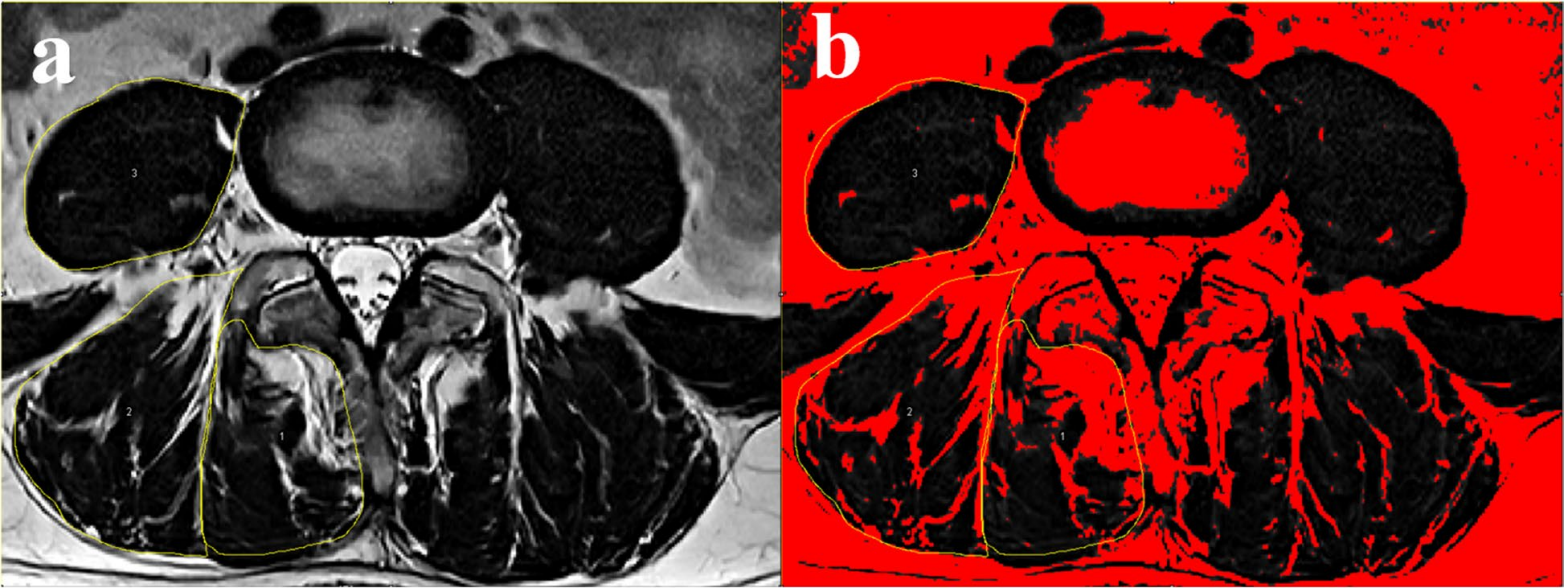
a Measurements of paraspinal muscular parameters on axial T2-weighted MRI (a 62-year-old woman). Regions of multifidus (1), erector spinae (2) and psoas muscle (3) at L4 level were outlined by yellow lines. For psoas muscle, only functional muscle was outlined. b Thresholding technique to highlight fatty area (red area)
